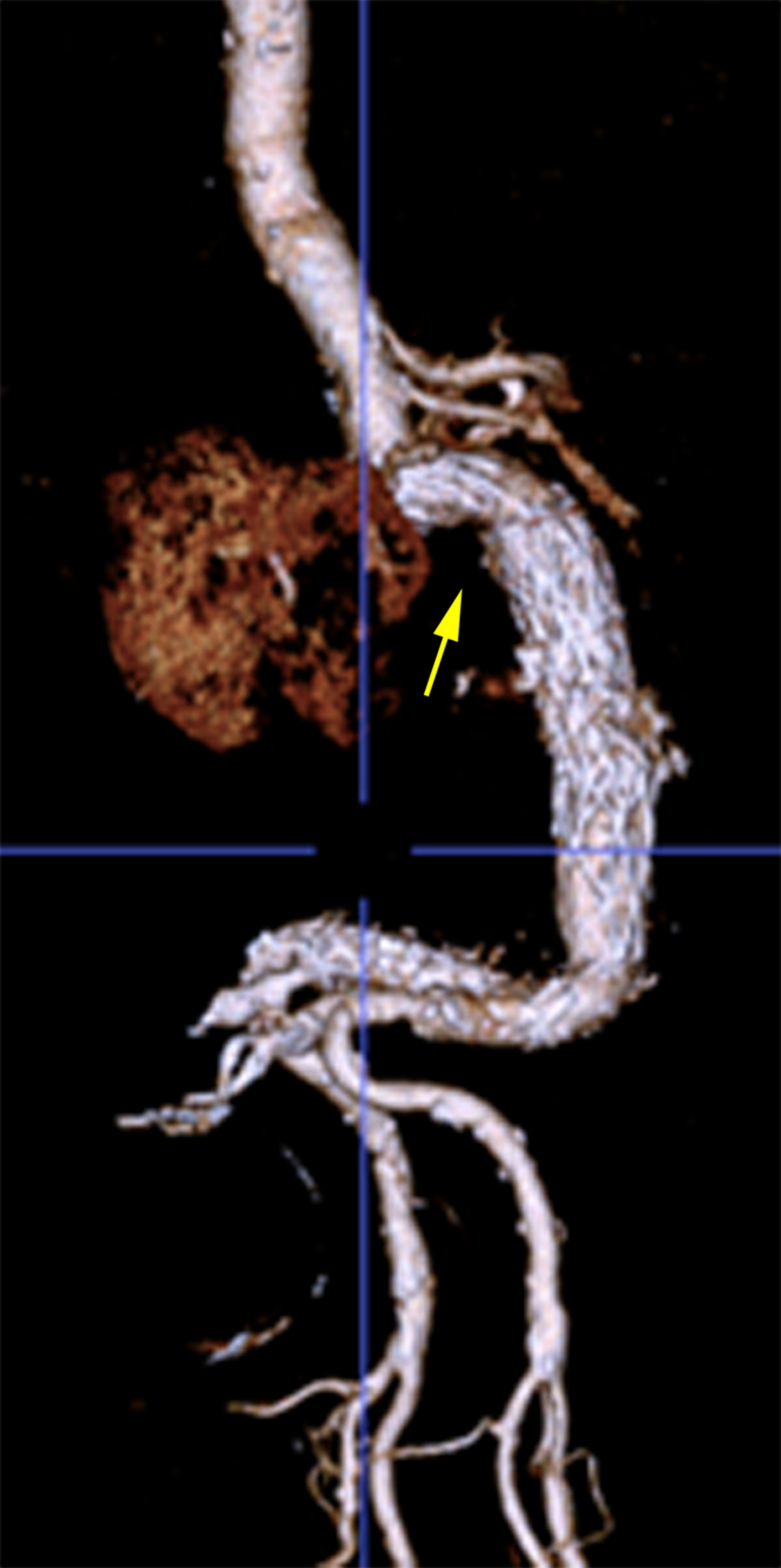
One-year follow-up CTA 3D reconstruction (sagittal orientation) showing a 90-degree neck angle and an intact stent graft CTA: computed tomography angiography; 3D: three-dimensional
